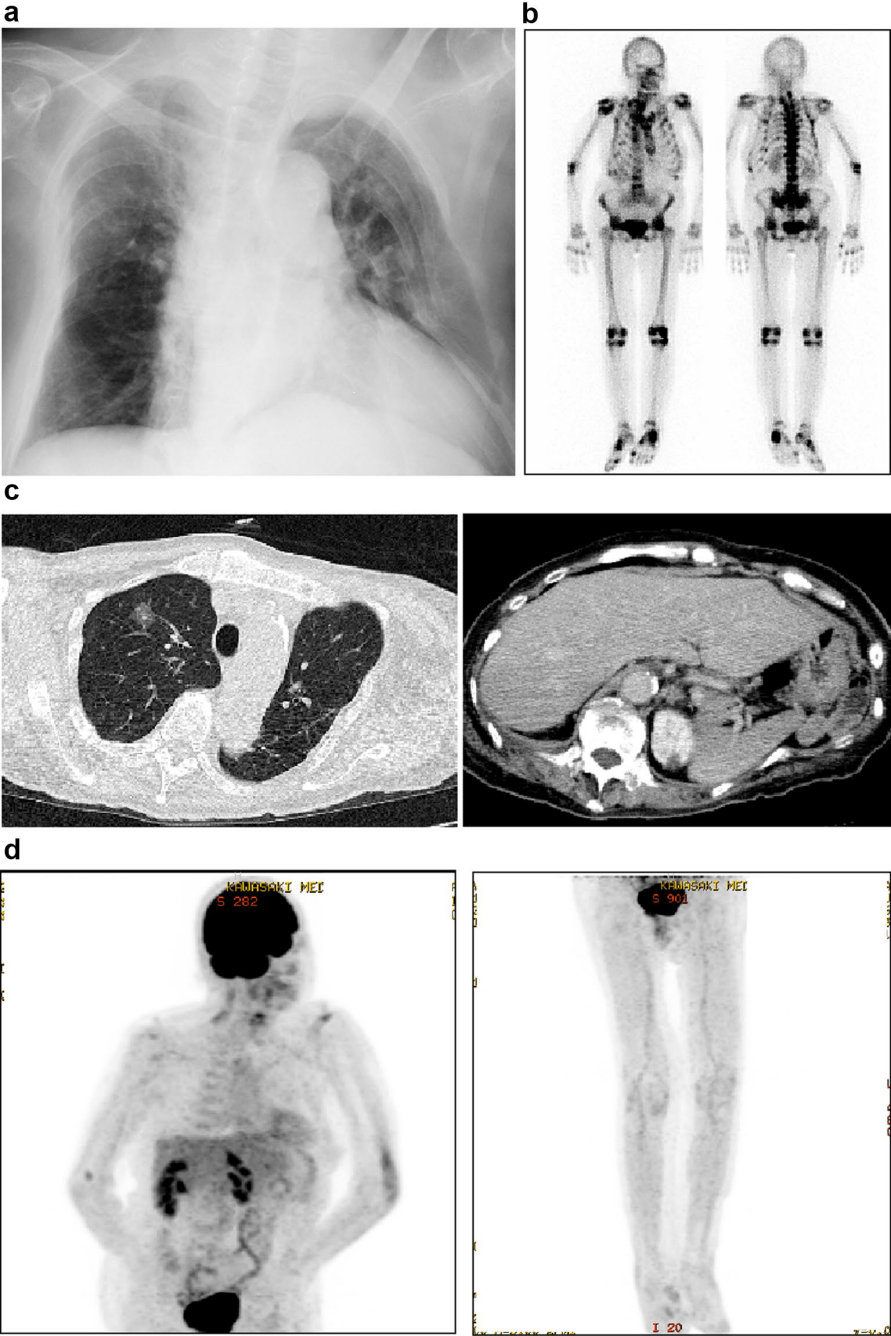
a Chest X-ray. There are remnants of multiple rib fracture, marked deformation of the thorax, scoliosis and cardiomegaly. b Bone scintigraphy. Abnormal accumulation is observed in ribs on both sides, limb joints. These are the findings after multiple fractures in the body. c Computer tomography (CT). There is sciatic fracture on both sides, multiple rib fracture, vertebral compression fracture and marked deformation in the thorax. Neoplastic lesion is not observed in bone and soft tissues. There is localized frosted glass shade in upper right lung field. d FDG-PET/CT. There is no abnormal accumulation in the whole body, although slight accumulation is observed in thoracolumbar spine and upper limb joint probably due to arthritis
